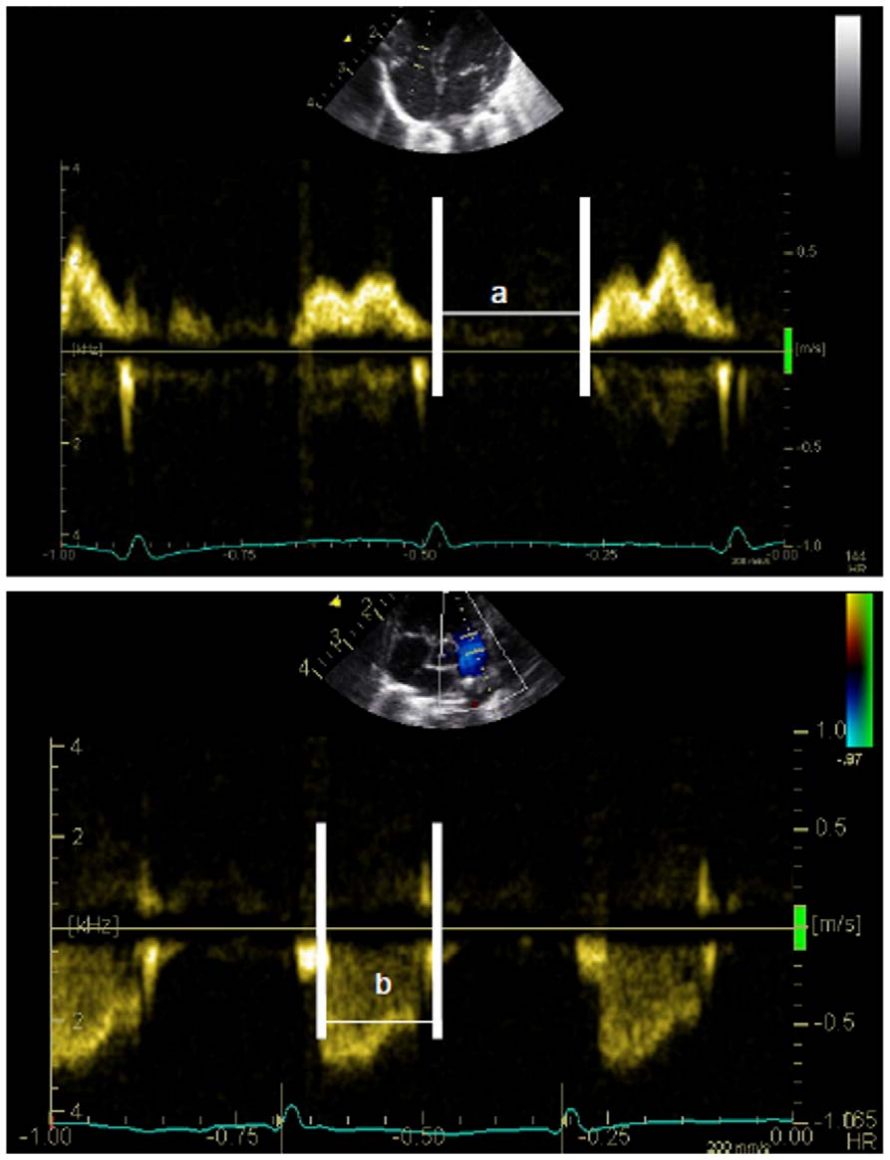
Doppler methodology. Measurement of the myocardial performance index by pulsed Doppler in the right ventricle capturing inflow and outflow. The “a” interval is the time from tricuspidal closing to opening, and the “b” interval is the ejection time in milliseconds.
